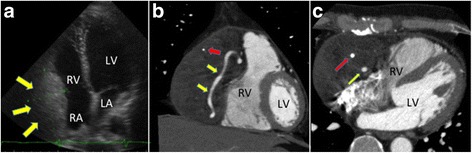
Diagnostic images. a Echocardiogram, demonstrating a hyperechoic mass (*yellow arrows*) adjacent to the RV. b, c Coronal (b) and transverse (c) coronary computed tomography scans, demonstrating a mass compressing the RV. The *red* and *yellow arrows* indicate calcification and the coronary artery, respectively. Abbreviations: *LA* left atrium, *LV* left ventricle, *RA* right atrium, *RV* right ventricle
